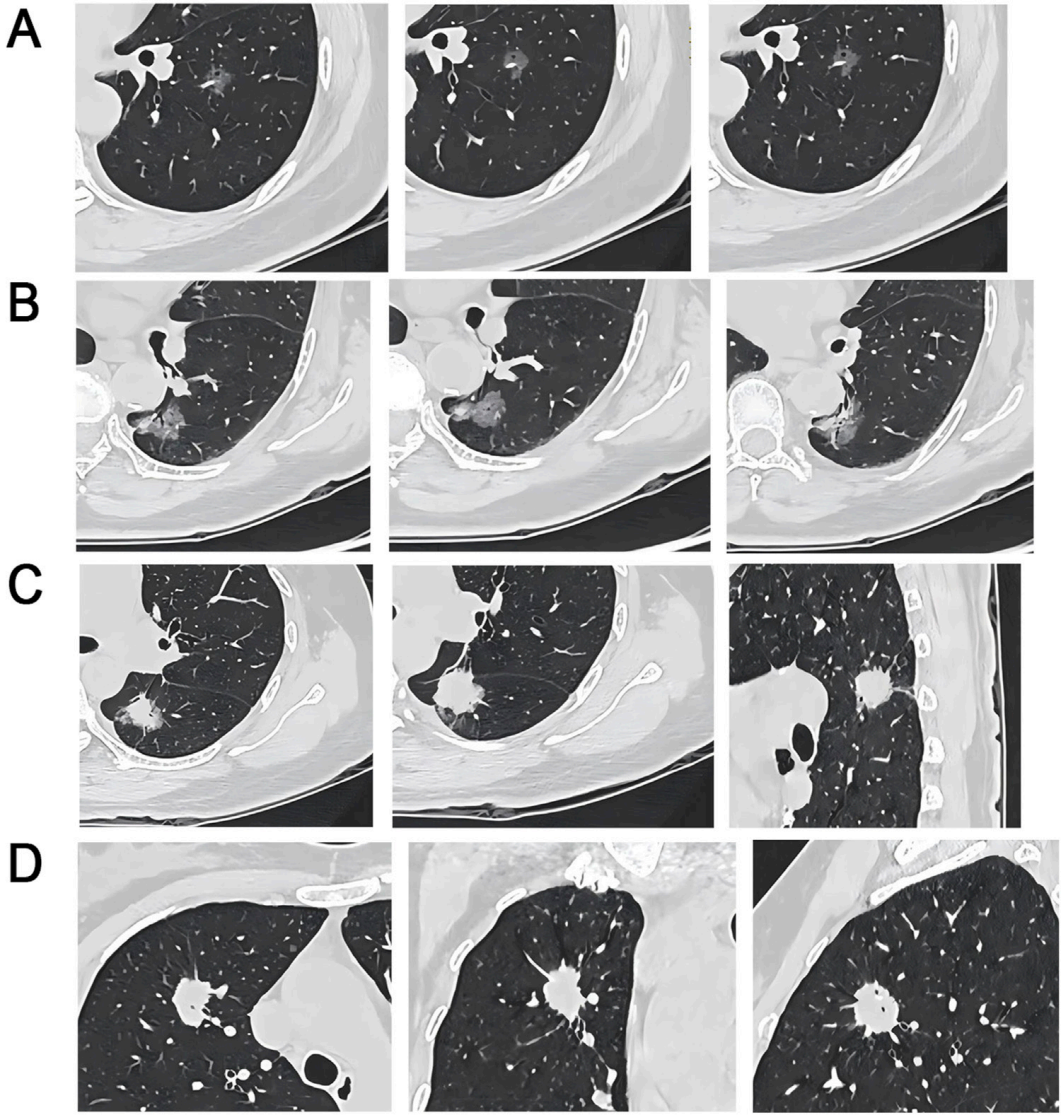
CT Imaging Characteristics of EGFR Wild-Type and ALK-Positive/Negative Lung Nodules (A) EGFR wild-type patient, 61-year-old female, pure GGO in the left lower lobe with vacuole, lobulation, spiculation, and PIS; (B) Circular EGFR wild-type, 75-year-old female, mixed GGO in the left lower lobe with pleural indentation, bronchial, vacuole, lobulation, and PIS; (C) ALK-negative, 56-year-old female, sub-solid nodule in the left lower lobe with bronchial, pleural indentation, spiculation, and deep lobulation signs; (D) ALK-positive, 51-year-old female, solid nodule in the right upper lobe with vacuole and spiculation signs.
